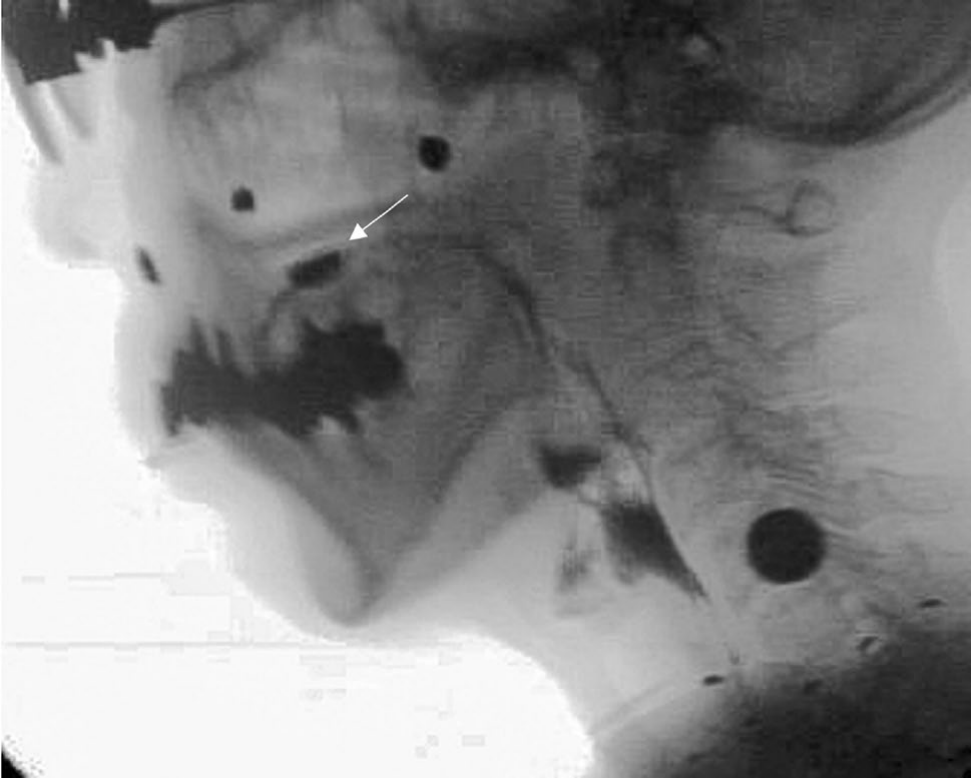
Radiograph, lateral view, showing pill residue in the mouth. The arrow indicates the pill residue.
